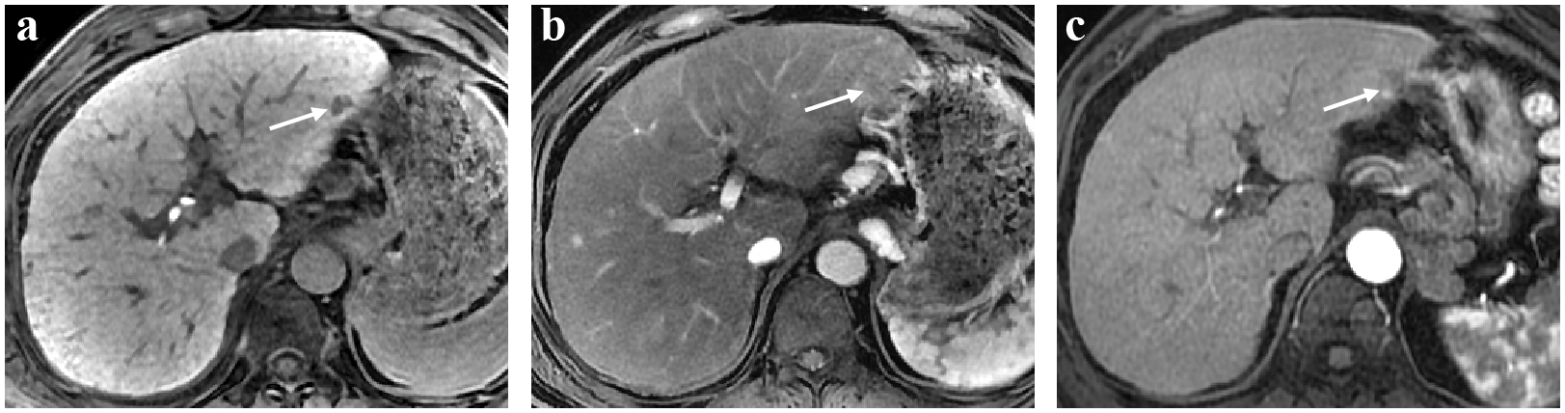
(A) Hepatobliiary phase (HBP) of gadoxetic acid-enhanced MRI, showed a hypointense nodule (arrow), which was considered as a liver nodule that showed a signal that was lower than that in the surrounding normal liver parenchyma. (B) Arterial phase of gadoxetic acid-enhanced MRI, showed a hypovascular nodule (arrow), which was defined as a liver nodule showing no evidence of arterial phase enhancement compared to that in the adjacent normal liver parenchyma. (C) Arterial phase of the follow-up MRI, showed vascularization development, which was defined as enhancement of the HHN.
